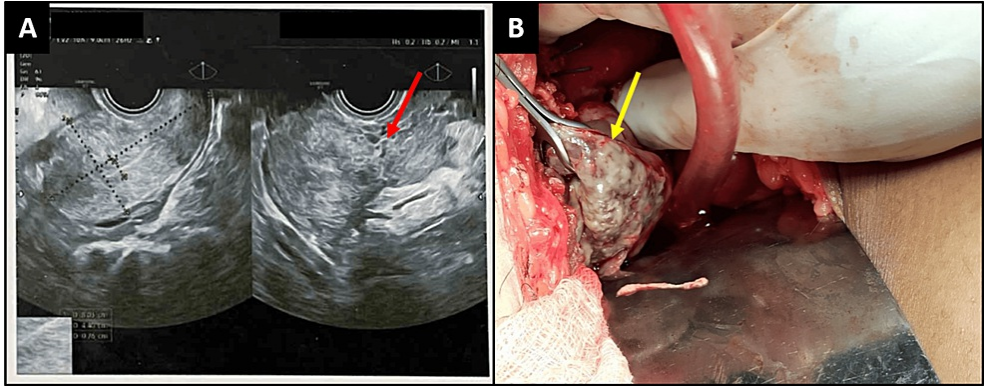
Ultrasound of the left adnexa shows the presence of heteroechoic mass lesion measuring 61x40x70 mm with internal necrosis (A). The intraoperative image reveals the left ruptured ovarian abscess measuring 6 cm x 7 cm (B).
